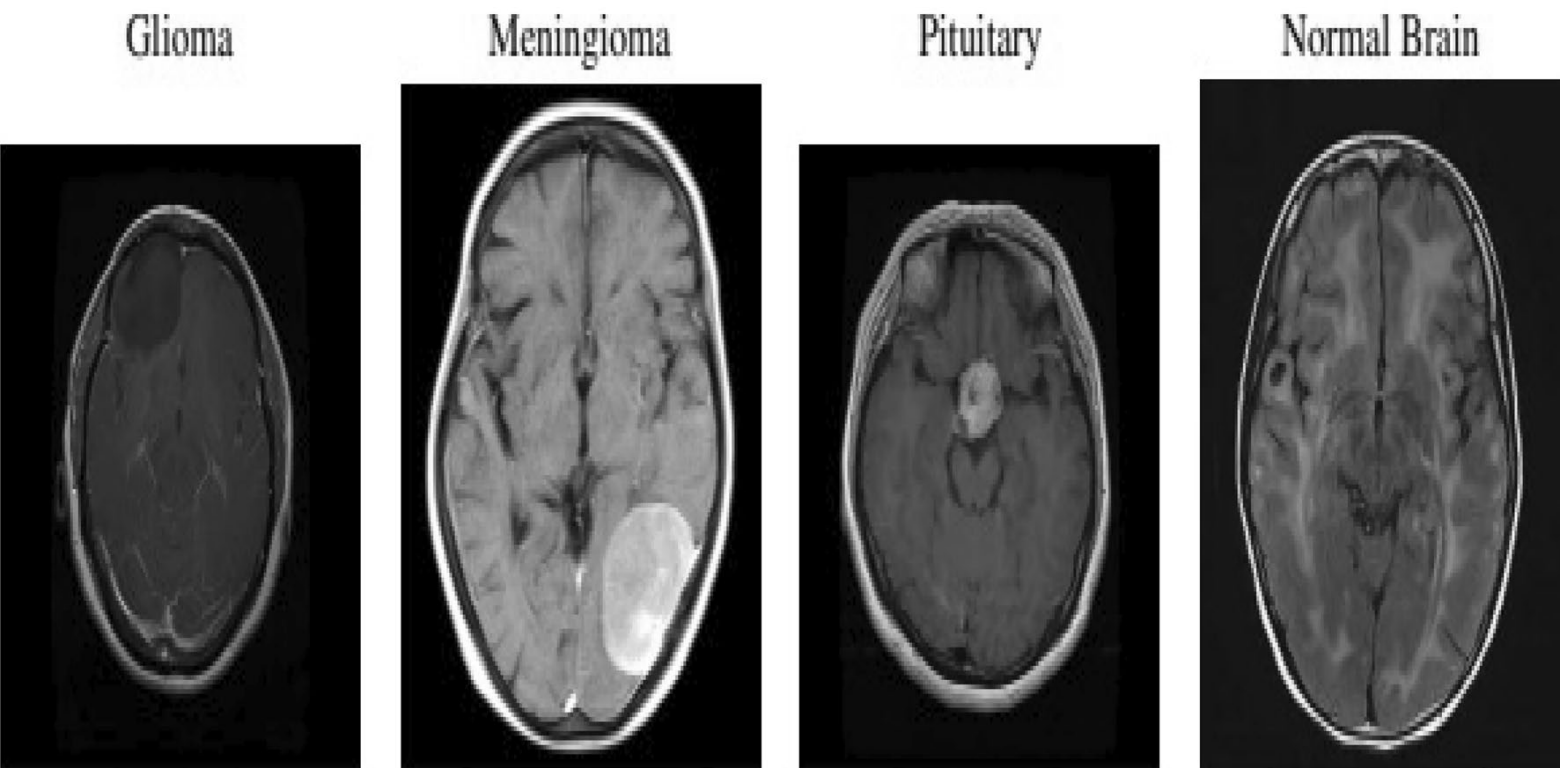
Standardized MRI images of various tumor types and orientations.
